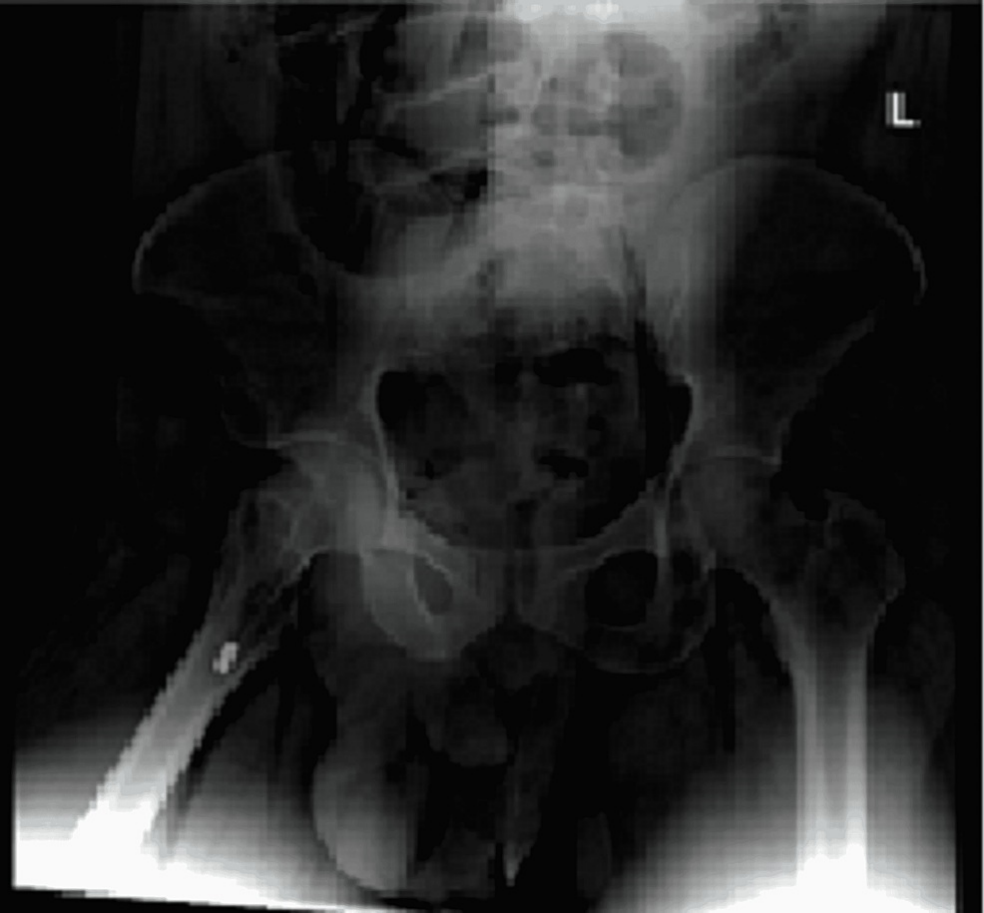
Forensic postmortem pelvic and upper part of lower limbs radiograph L: left.
